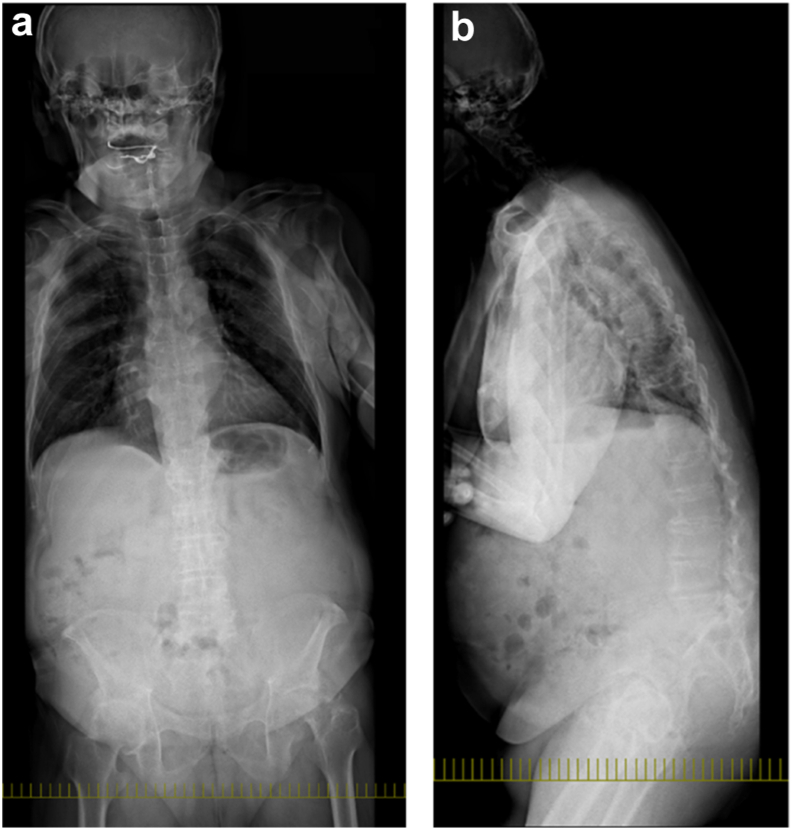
Preoperative full-spine radiograph. (a) Anteroposterior view and (b) Lateral view.
